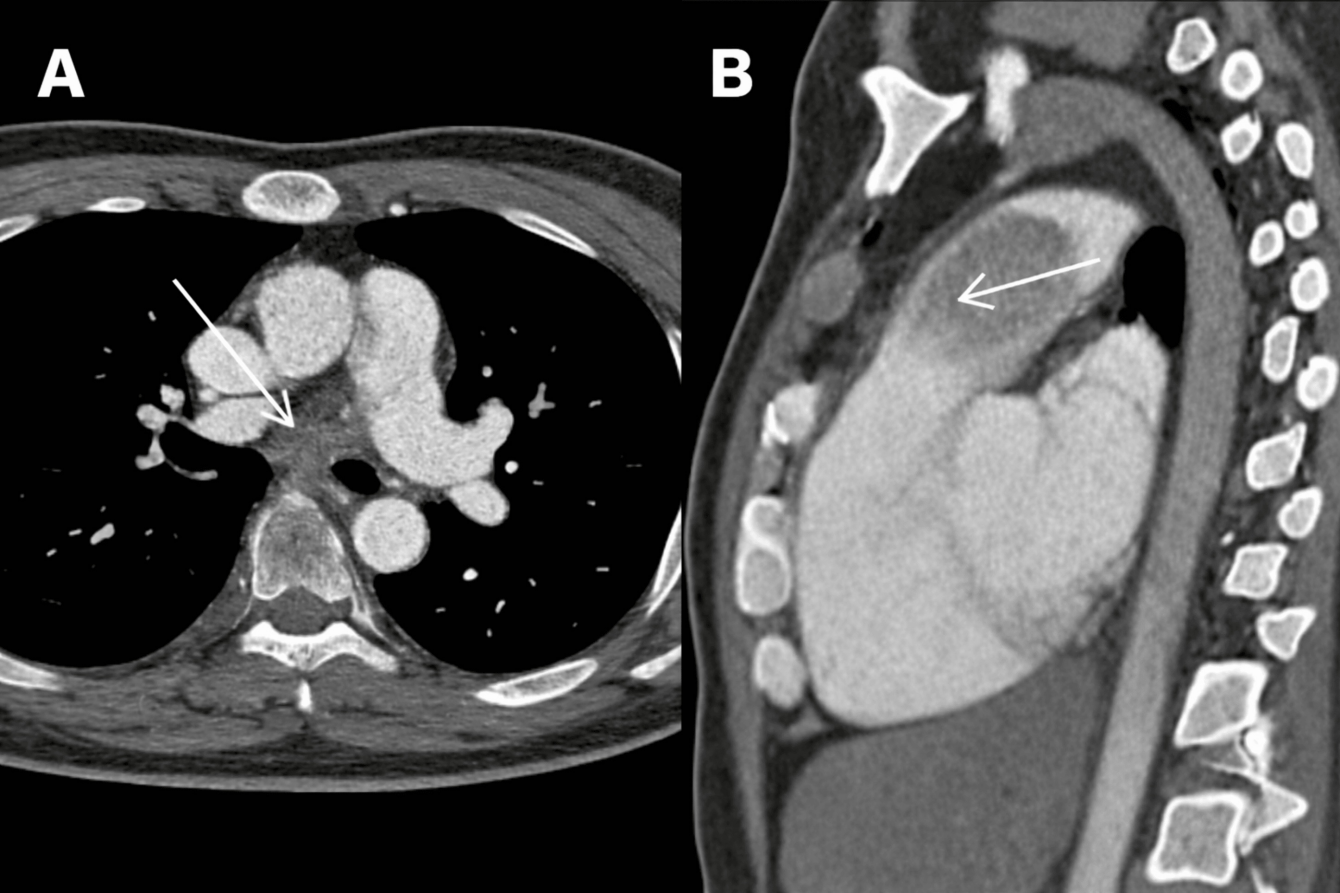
Contrast-enhanced computed tomography pulmonary angiography (A) Axial image showing an intraluminal soft-tissue mass (arrow) in the main pulmonary artery causing near-complete obstruction. The lesion demonstrates a broad-based attachment to the arterial wall, which is atypical for thrombus. (B) Sagittal image again demonstrating the mass (arrow) extending longitudinally along the main pulmonary artery toward the right ventricular outflow tract.
